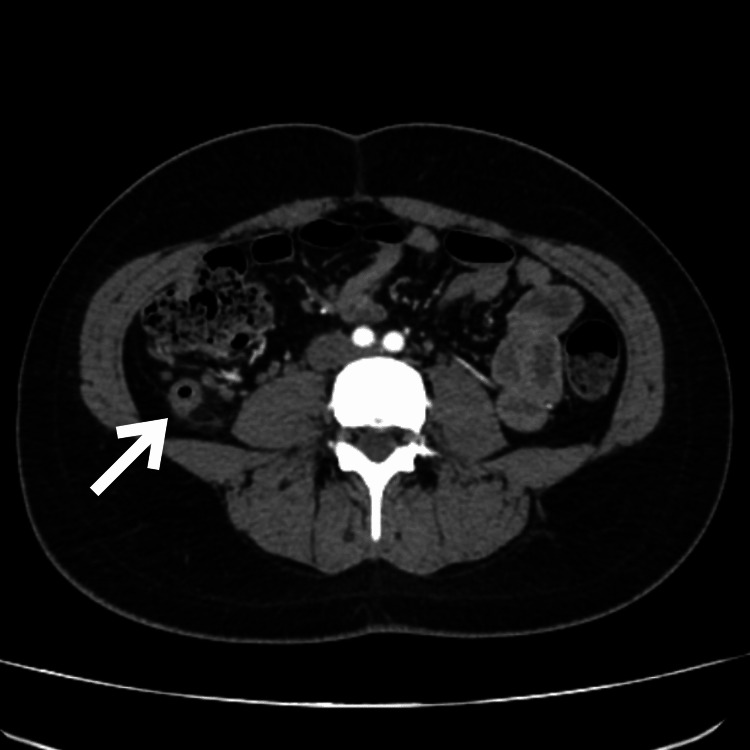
Computed tomography scan showing a dilated appendix, axial view (white arrow).
